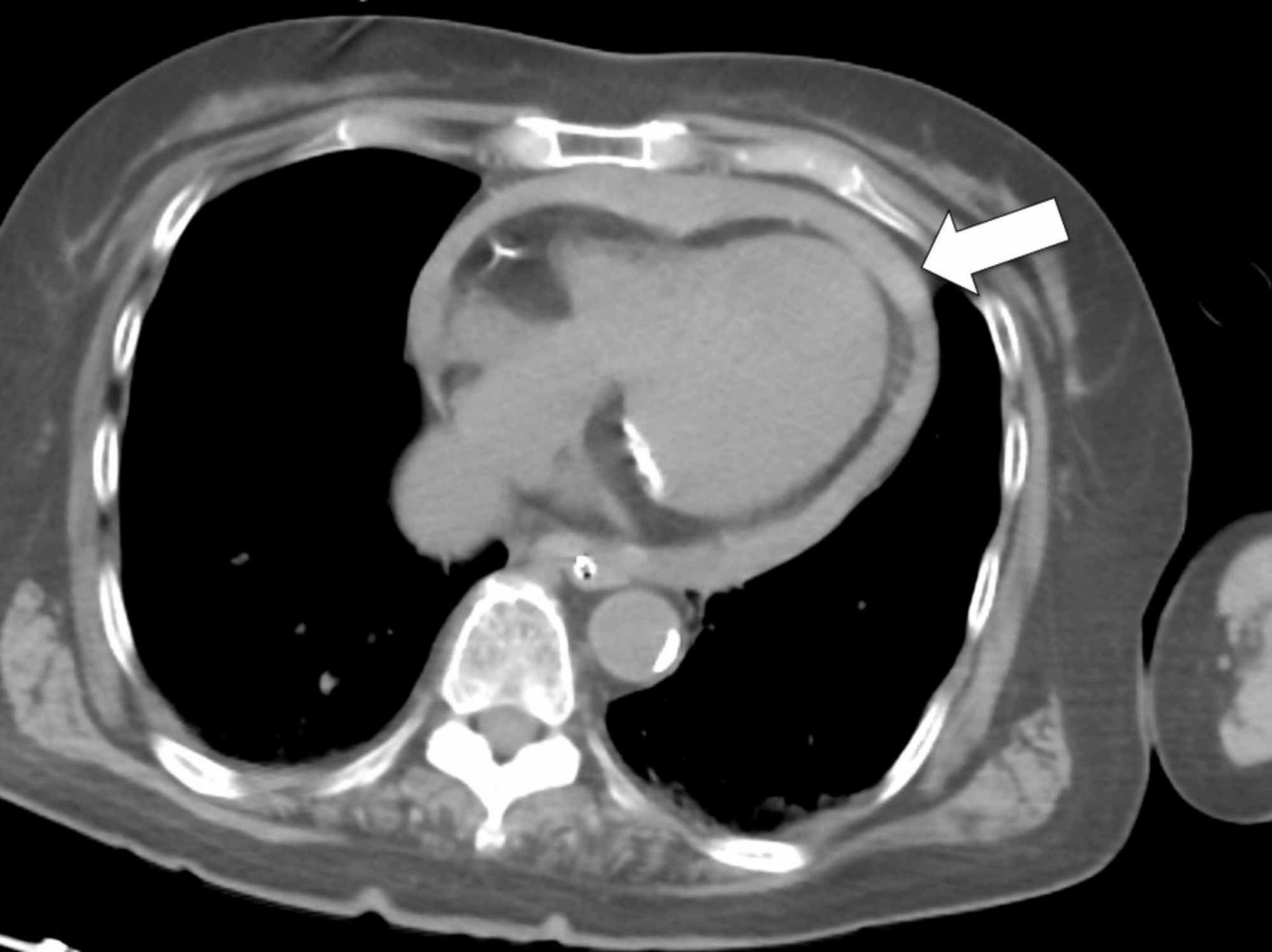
Computed tomography (CT) on arrival CT demonstrated cardiac tamponade (arrow).
